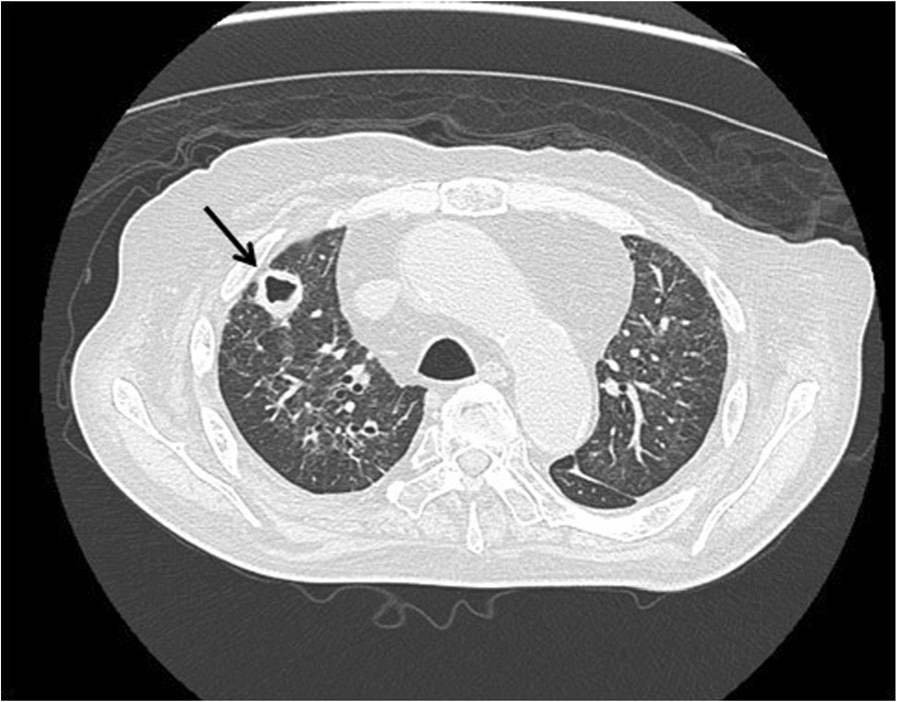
Hollow nodules seen on chest CT
